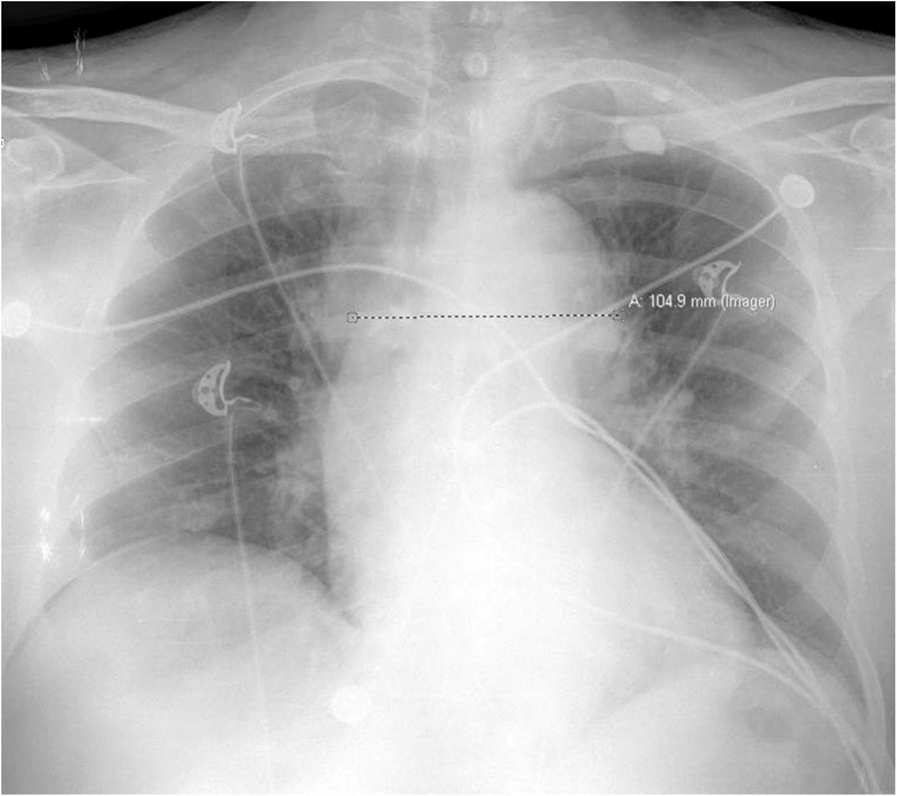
Chest radiography, upright, demonstrating a widened mediastinum at 10.5 cm
